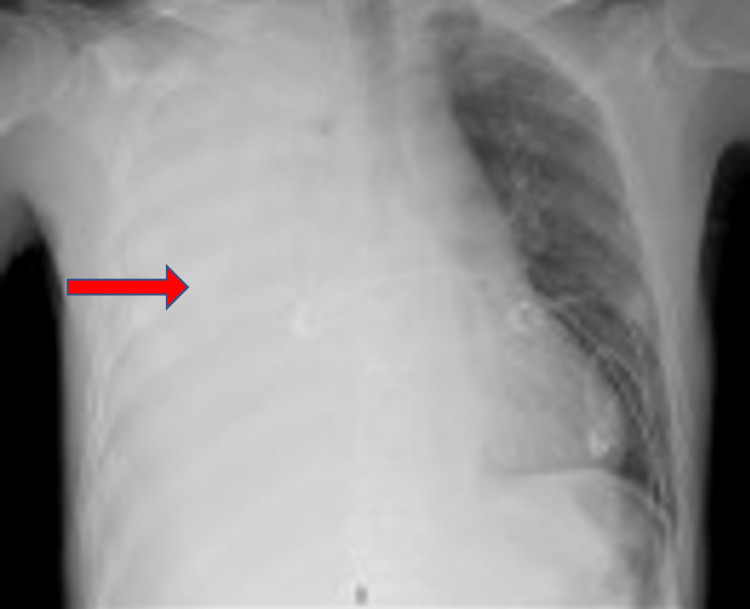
Portable (AP) chest X-ray shows right-sided pleural effusion with near-complete opacification of the right hemithorax shown by the red arrow.
